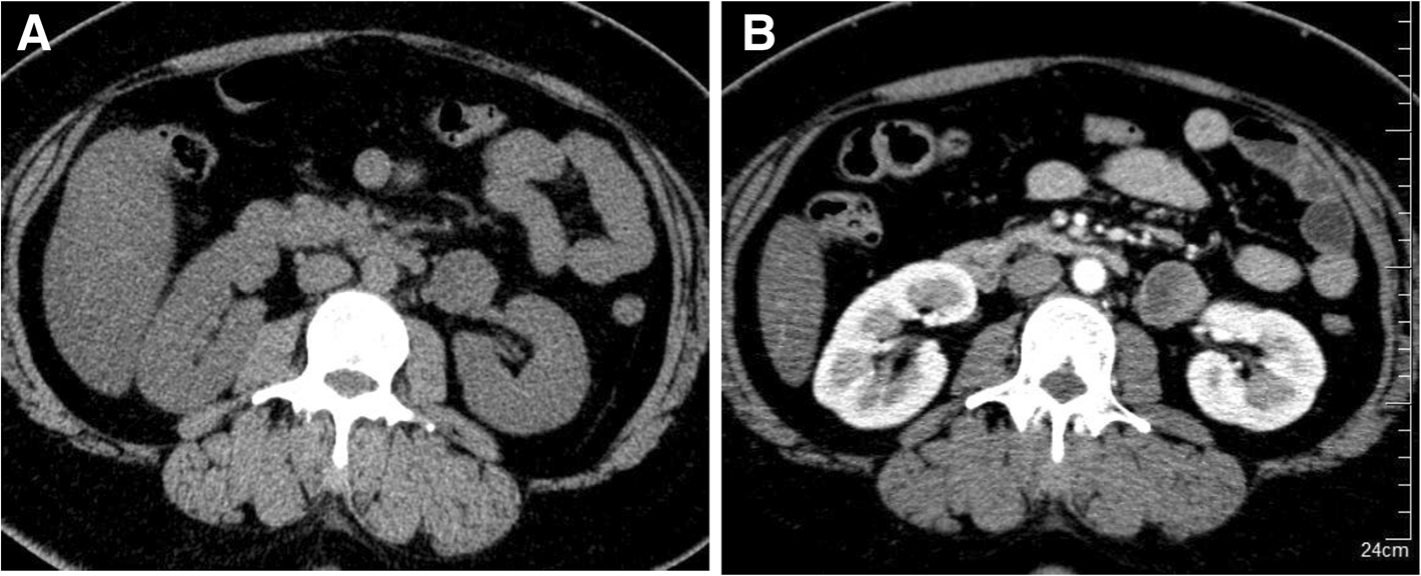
Abdominal computed tomography findings. Plain computed tomography showed a homogenous round tumor of 4×3×2cm in size (A) and weak enhancement in the peripheral area of the tumor (B).
